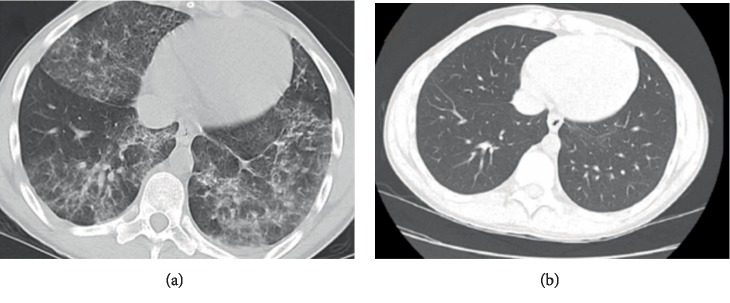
(a) CT scan of the chest without contrast done prior to steroids demonstrating bilateral, multilobar diffuse interstitial and mixed infiltrates evident as “honey combing” and “ground glass” appearance, with well-defined fissure line showing that the infiltrate do no cross between lobes. (b) HRCT scan of the chest post steroid therapy showing resolution of the previously evident diffuse opacity. The scans are 5 weeks apart.
